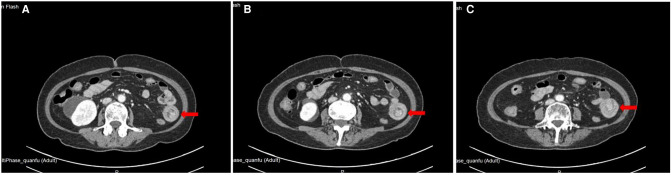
Location of small intestinal intussusception in abdominal enhanced CT.
